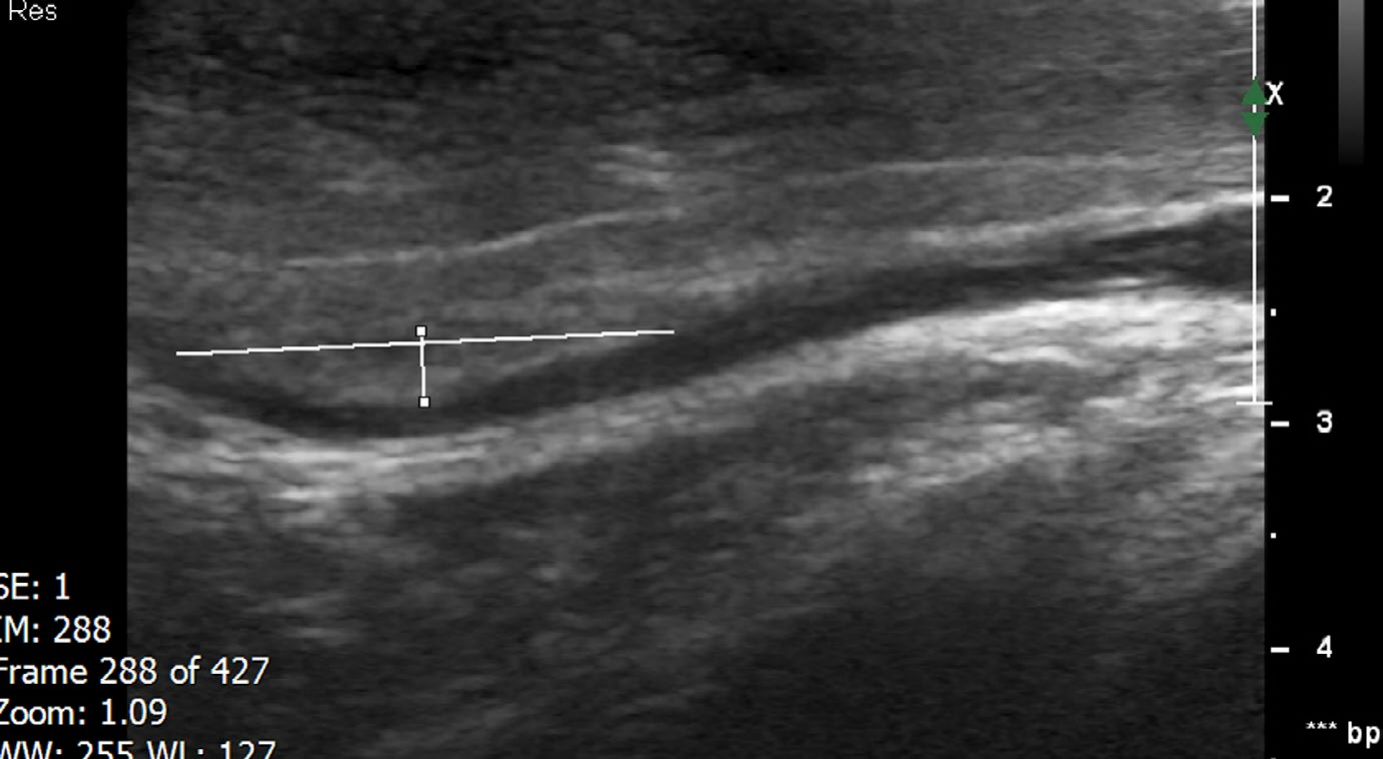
Two Months after starting the treatment. Ultrasound of the deep dorsal penile vein revealed no evidence of acute thrombosis. The vein is seen with normal diameter. At the proximal part of the vein, the dorsal wall is thickened and an old partial thrombosis measured 26 × 2.4 mm is noted. Other parts of the vein showed normal velocity with no other partial thrombosis. The vein got expanded with valsalva maneuver. Patient had a little pain with the pressure of ultrasound probe at the site of the partial thrombosis
